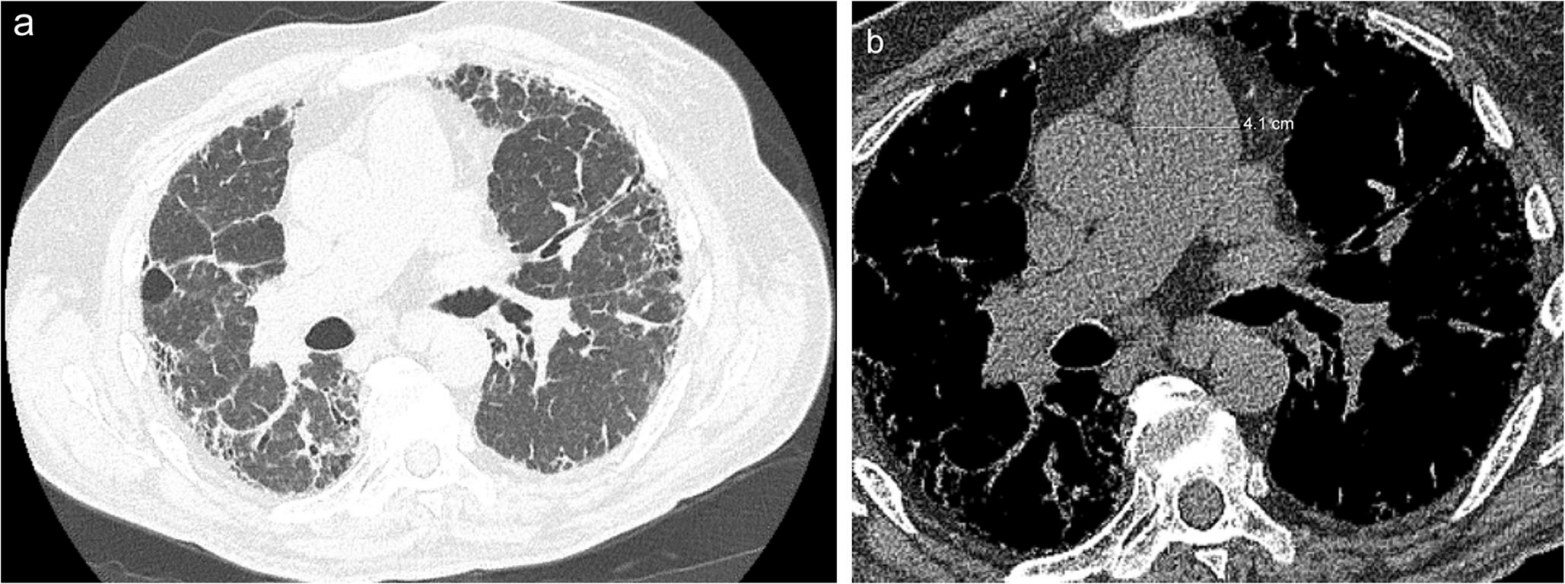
Pulmonary hypertension in a patient with idiopathic pulmonary fibrosis. a Axial CT image (lung window) shows basal and peripheral predominant fibrotic disease with reticulation and honeycombing extending to the subpleural surface, as well as traction bronchiectasis, consistent with usual interstitial pneumonia pattern of fibrosis in a patient with idiopathic pulmonary fibrosis. b Axial CT image shows dilatation of the main pulmonary artery, measuring up to 4.1 cm in maximal diameter
